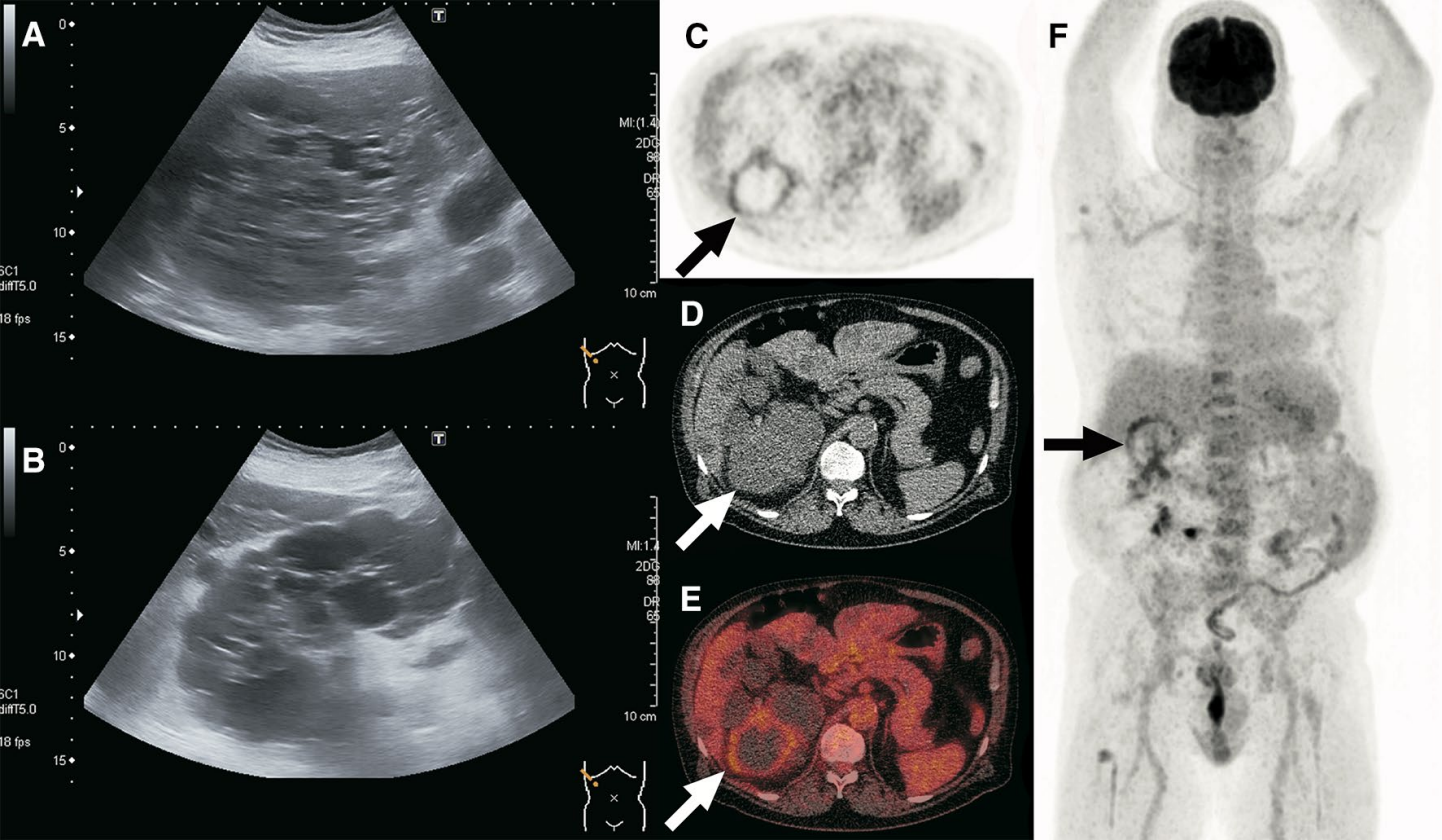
A 61-year-old man with ADPKD and unilateral renal agenesis was admitted to the hospital because of a possible urinary tract infection. He presented with complaints of cold shivers, pollakiuria, stranguria, lower abdominal pain and cloudy urine. Blood tests showed a CRP level of 363 mg/L and a white blood cell count of 6.6 × 10^9^/L, while a urine culture tested positive for *Escherichia coli*. The patient’s clinical condition improved under ciprofloxacin treatment, but his CRP level remained high 5 days later at 154 mg/L. Because of the possibility of pyelonephritis or cyst infection, ultrasound was ordered. Ultrasonographic images of the liver (a) and kidney (b) showed multiple cysts, but no hydronephrosis and no potential site of infection. Subsequently, FDG-PET/CT was performed. Axial FDG-PET (c), low-dose CT (d), fused FDG-PET/CT (e), and coronal maximum intensity projection FDG-PET (f) showed pathologic FDG uptake of the wall of a cyst in the right kidney (arrows), consistent with cyst infection. No pathologic FDG-avid foci were detected elsewhere. The patient was discharged 1 day after the FDG-PET/CT scan in good clinical condition, where he continued his course of ciprofloxacin for 10 more days
